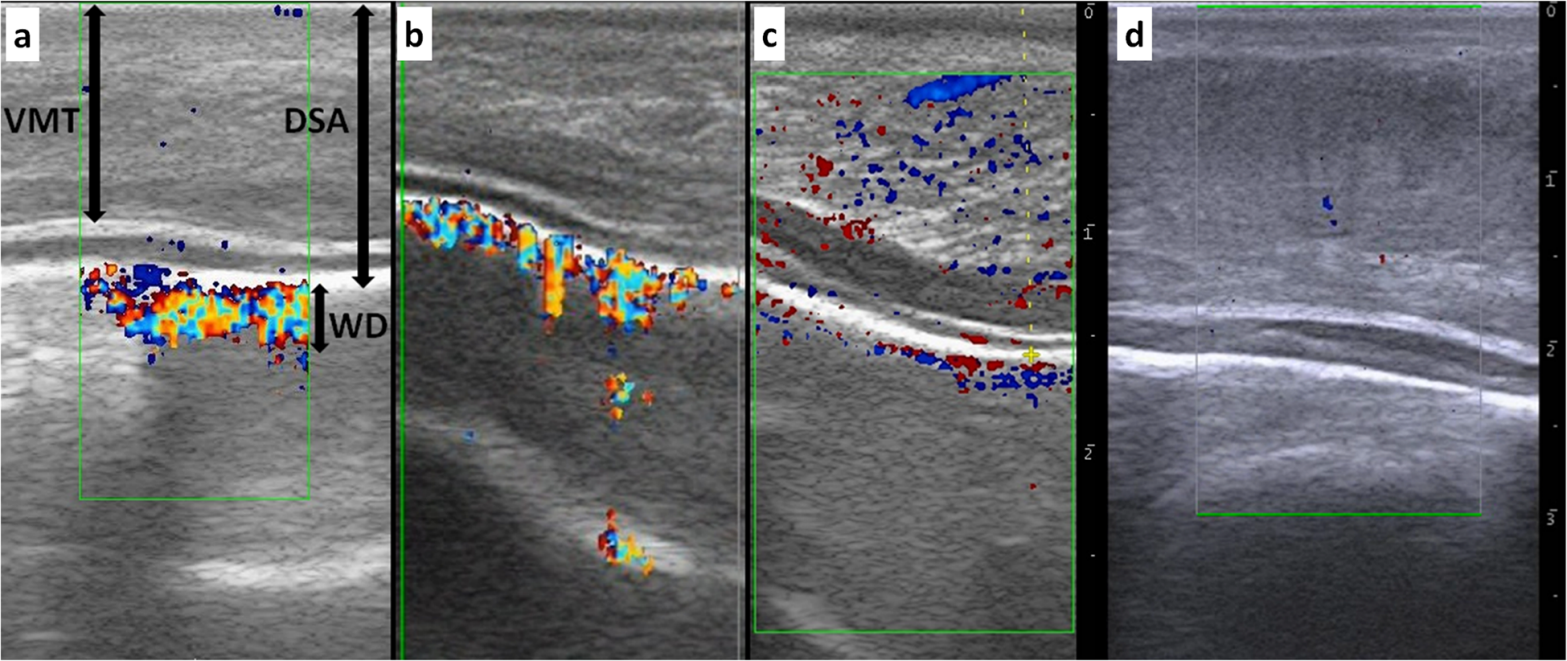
Sagittal ultrasonographic images of the tongue ventral surfaces in three of five healthy calves (a-c), and the affected case (d). a,b Normal ventral lingual structures exhibit 1) muscular layers in which the echogenic muscular fibers are regularly aligned, 2) two hyperechoic lines, which include homogenous hypoechoic structure, and 3) the deep lingual artery running along the distal hyperechoic line. Lingual structures deeper than the hyperechoic lines disappear due to a reverberation artifact. Rich blood flow is noted in the deep lingual artery on the Color-flow Doppler ultrasonograms. VMT: ventral muscular thickness. DSA: distance between the ventral surface of the tongue and the deep lingual artery. WD: width of the colored region on Doppler image of the deep lingual artery. c Blood flow is weakened into the deep lingual artery, but is comparatively rich in the intramuscular vessels on the Color-flow Doppler ultrasonogram. d The ventral lingual muscular layers are thicker than that of the normal tongue, but retains the regular alignment of the echogenic muscular fibers. Blood flow is not evident into the deep lingual artery on the Color-flow Doppler ultrasonogram. Scale: 10 mm
